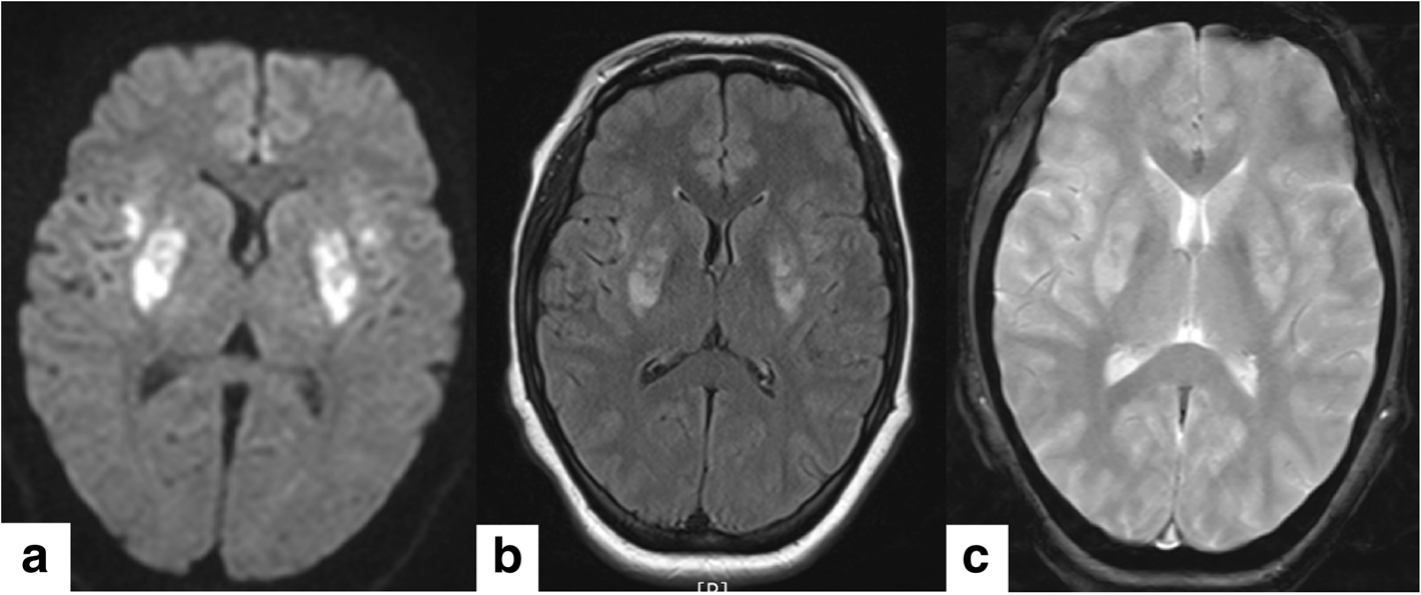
Brain magnetic resonance image (MRI) showed abnormal signal intensity in the bilateral putamen and anterior insular cortices. a diffuse restriction in the bilateral putamen and anterior insular cortices in diffusion weighted image, b high signal intensities in T2-weighted image on the same region, c: focal hemorrhage was not detected in susceptibility weighted image
